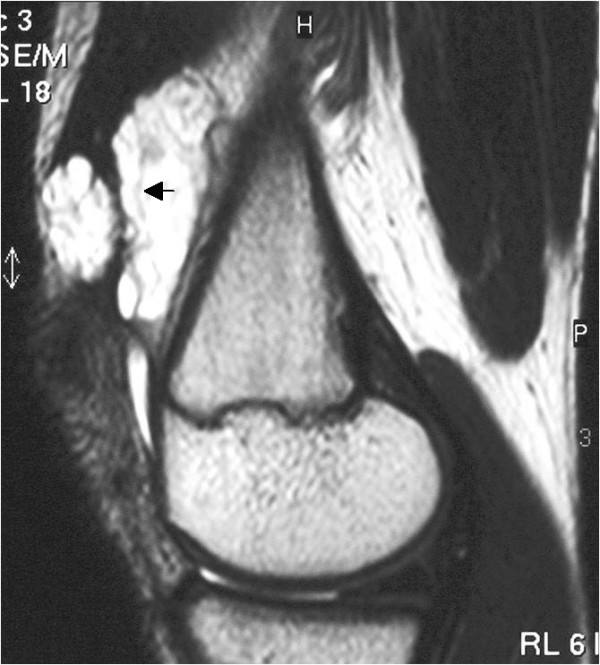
Sagittal T2-weighted fat suppressed image of the left knee showing thin fibrofatty septae of low signal intensity within the lesion (black arrow).
